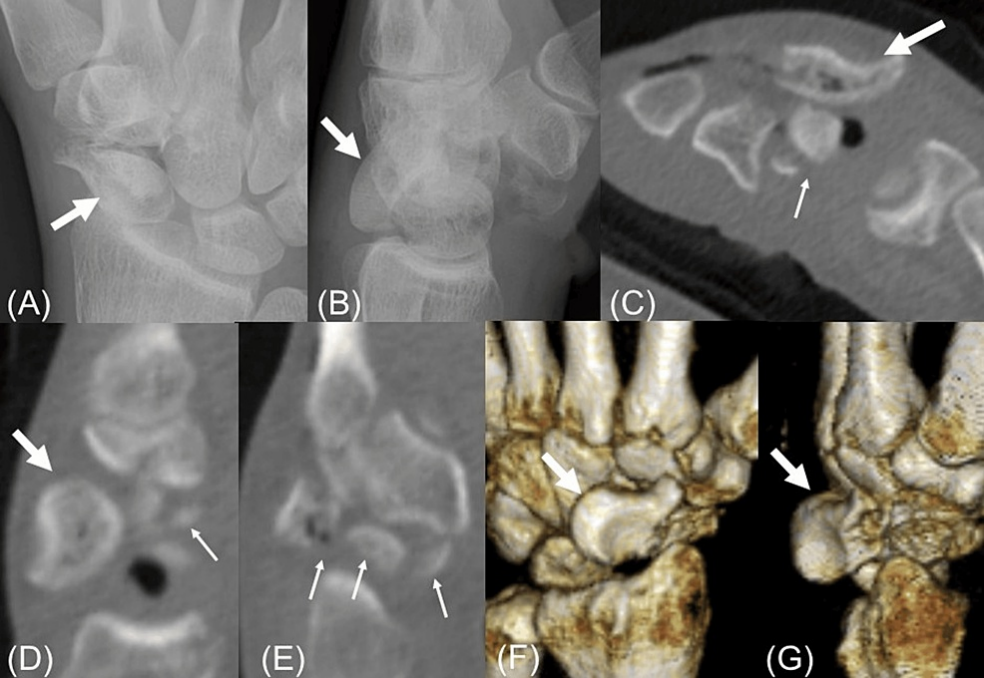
Initial radiographs and computed tomography scans. Initial radiographs and computed tomography (CT) scans show the scaphoid fracture and dorsal dislocation. The thick arrows show the proximal fragment of the scaphoid. The thin arrows show the distal fragment of the scaphoid. (A) Antero-posterior film of the left wrist shows a comminuted scaphoid fracture. (B) The lateral film of the left wrist shows dorsal dislocation of the proximal fragment of the scaphoid. (C-E) Axial and sagittal CT images show a comminuted scaphoid fracture with dorsal dislocation of the proximal fragment. (F, G) 3D CT reconstruction of the wrist in different views shows dorsal dislocation of the proximal fragment of the scaphoid.
